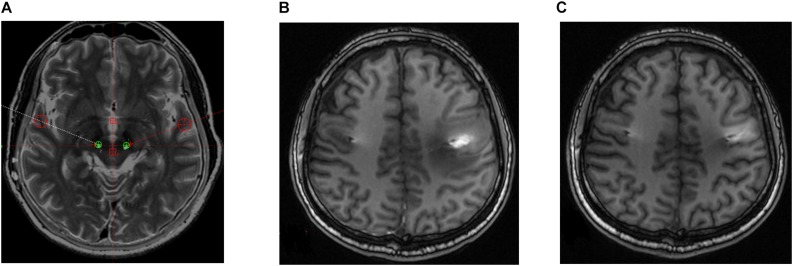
Postoperative brain magnetic resonance (MR) images in a patient who developed right paralysis due to encephaledema. (A) Position of electrodes. (B) MR image taken 1 week postoperatively. (C) MR image taken 8 weeks postoperatively.
